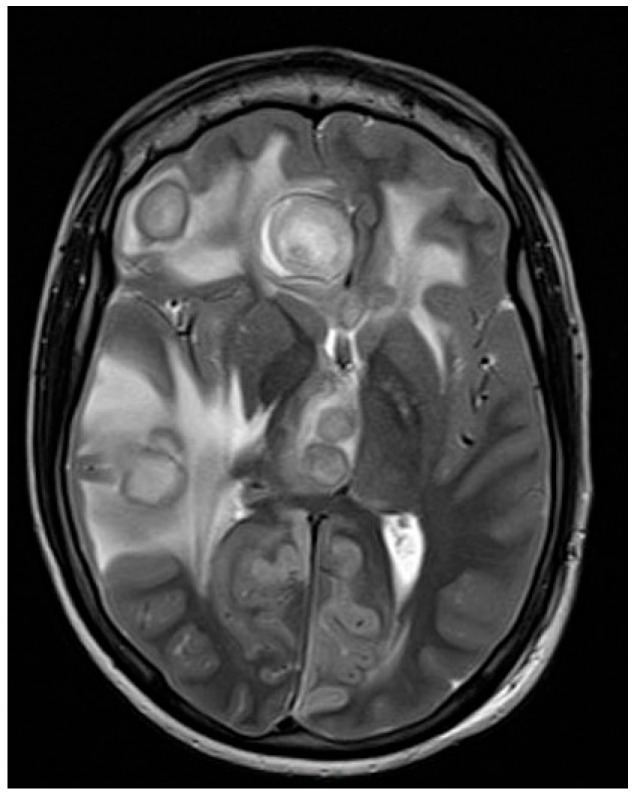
Baseline MRI, multiple focal lesions with a widespread bihemispheric distribution (right > left) and involvement of the right thalamus, accompanied by extensive vasogenic perilesional edema.
